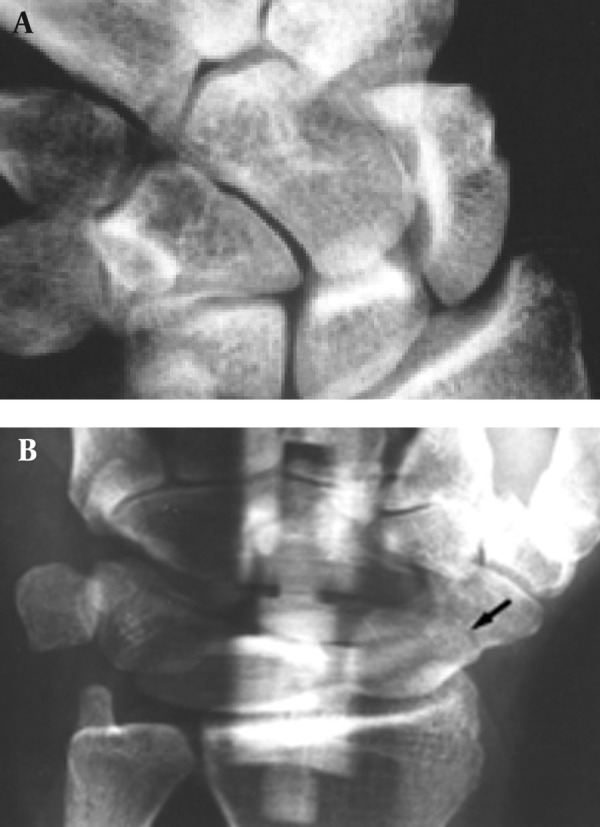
A 49-year-old woman with acute trauma of the wrist. Conventional and panoramic radiography were performed 1 week after injury. A, Conventional radiograph shows no evidence of fracture. B, Panoramic radiograph shows fracture line.
